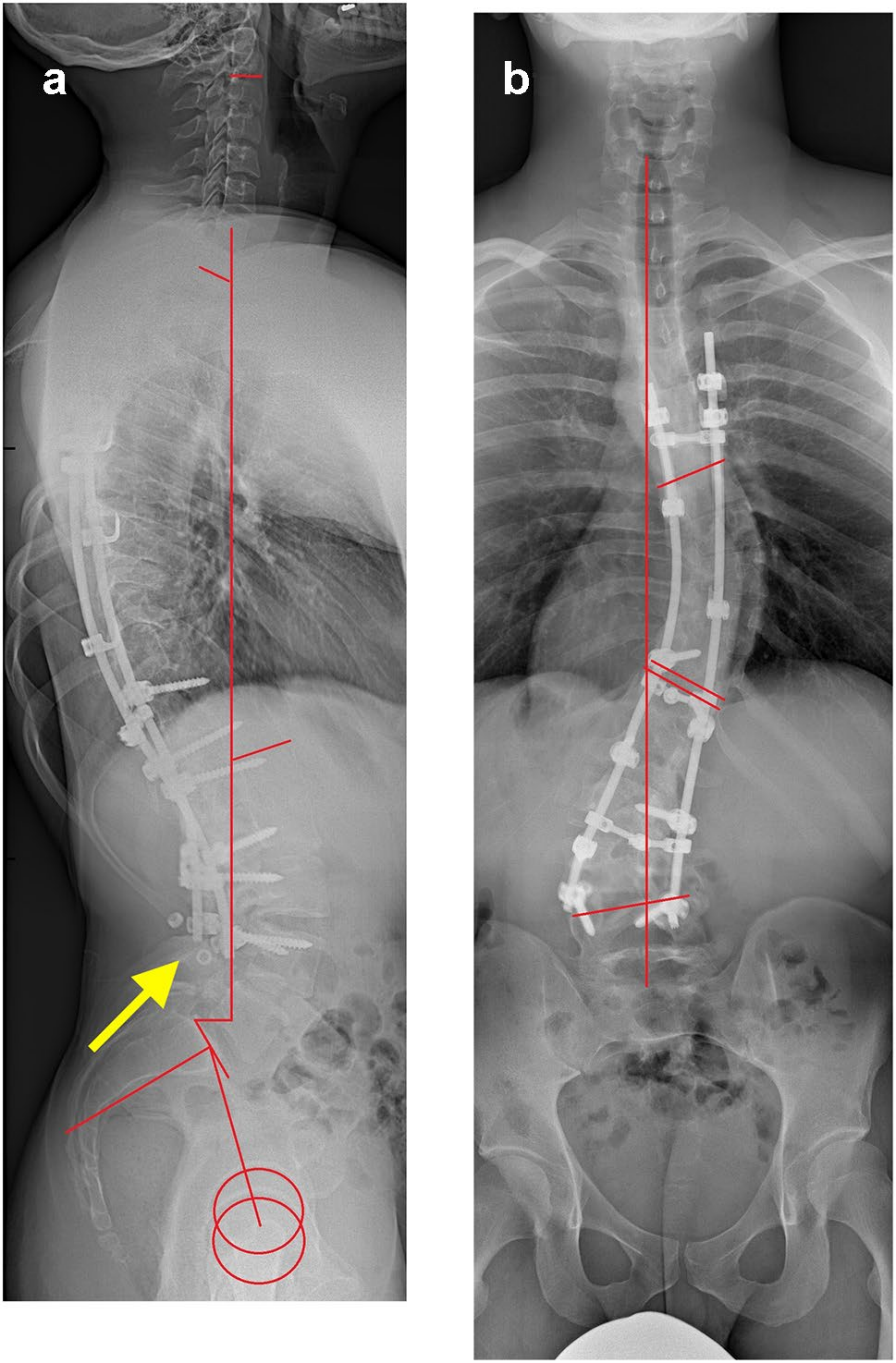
Anteroposterior and lateral radiographs of the patient’s spine. Thoracic Cobb angle, 47°; lumbar Cobb angle, 37°; central sacral vertical line, sagittal vertical axis (19 mm); L1–S1 lumbar lordosis, 70°; pelvic tilt, 12°; sacral slope, 52°; and T5–T12 thoracic kyphosis, 43° (red lines). Dislodged set screws are visible on the lateral projection (yellow arrow)
